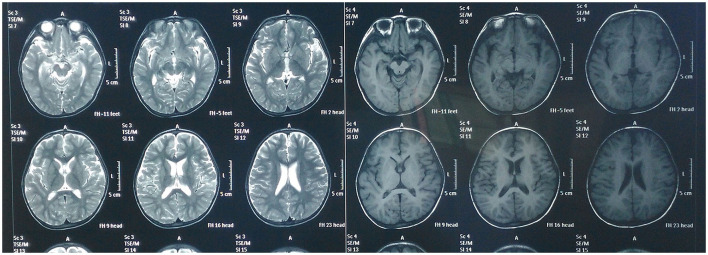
Magnetic resonance imaging of the brain after receiving Tongdu Xingshen acupuncture suggested mild brain atrophy and pachymeningeal enhancement associated to meningitis improved.
